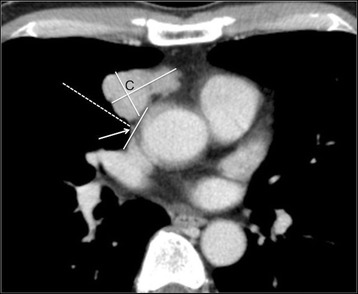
Axial CT detected a thymoma in the anterior mediastinum. The center of the tumor (C) was located on the medial side of the ipsilateral PCPV (arrow)
